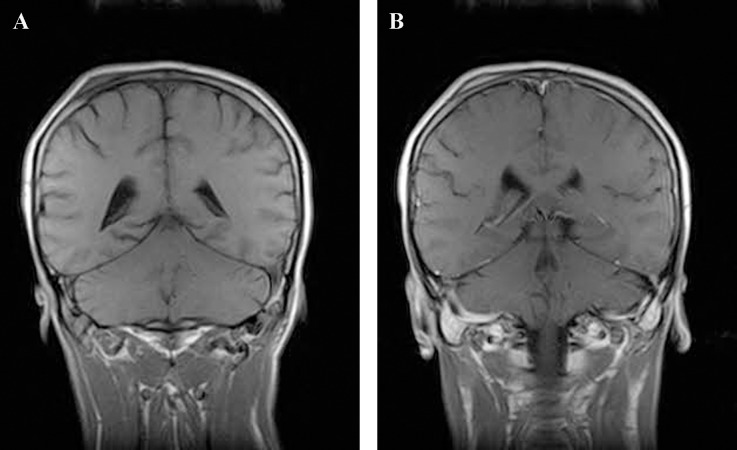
T1-weighted coronal MR image before (A) and after (B) gadolinium. A second small lesion with the same MR signal characteristics extending outward from the right parietal bone
